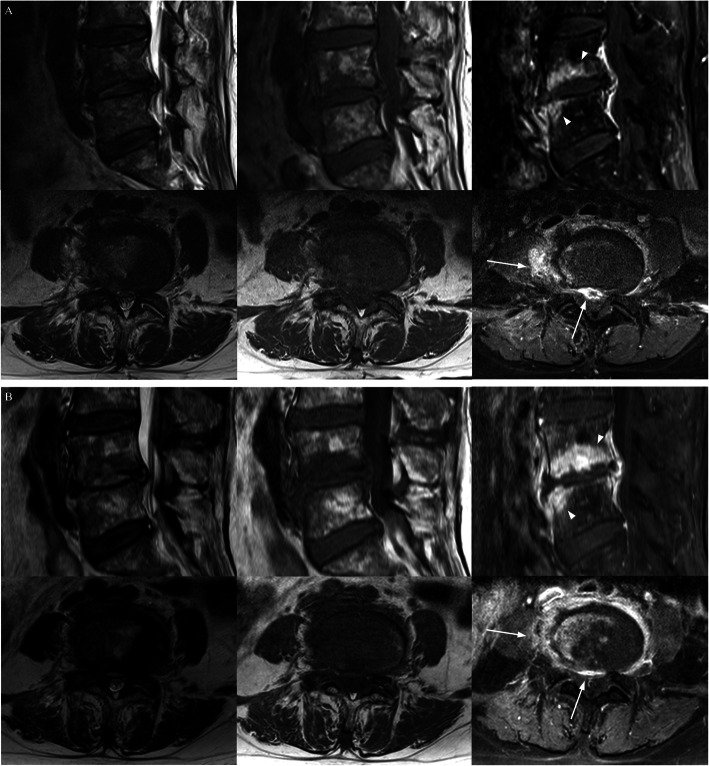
A patient with spondylodiscitis at L4–5 (culture (−), follow-up interval 44 days). T2-weighted (left), T1-weighted (middle), and contrast-enhanced fat suppressed T1-weighted images (right) of the spine. Comparing contrast-enhanced fat suppressed T1-weighted sagittal (upper) and axial (lower) images of baseline (a) and follow-up (b), bone findings worsened (arrow heads) and soft tissue findings improved (arrows). The disc height change was equivocal. CRP was resolved (24.4 to 3.41 mg/L [0.0–5.0]) and ESR was resolving (44 to 39 mm/hr. [0–15]) at baseline and follow-up, respectively
